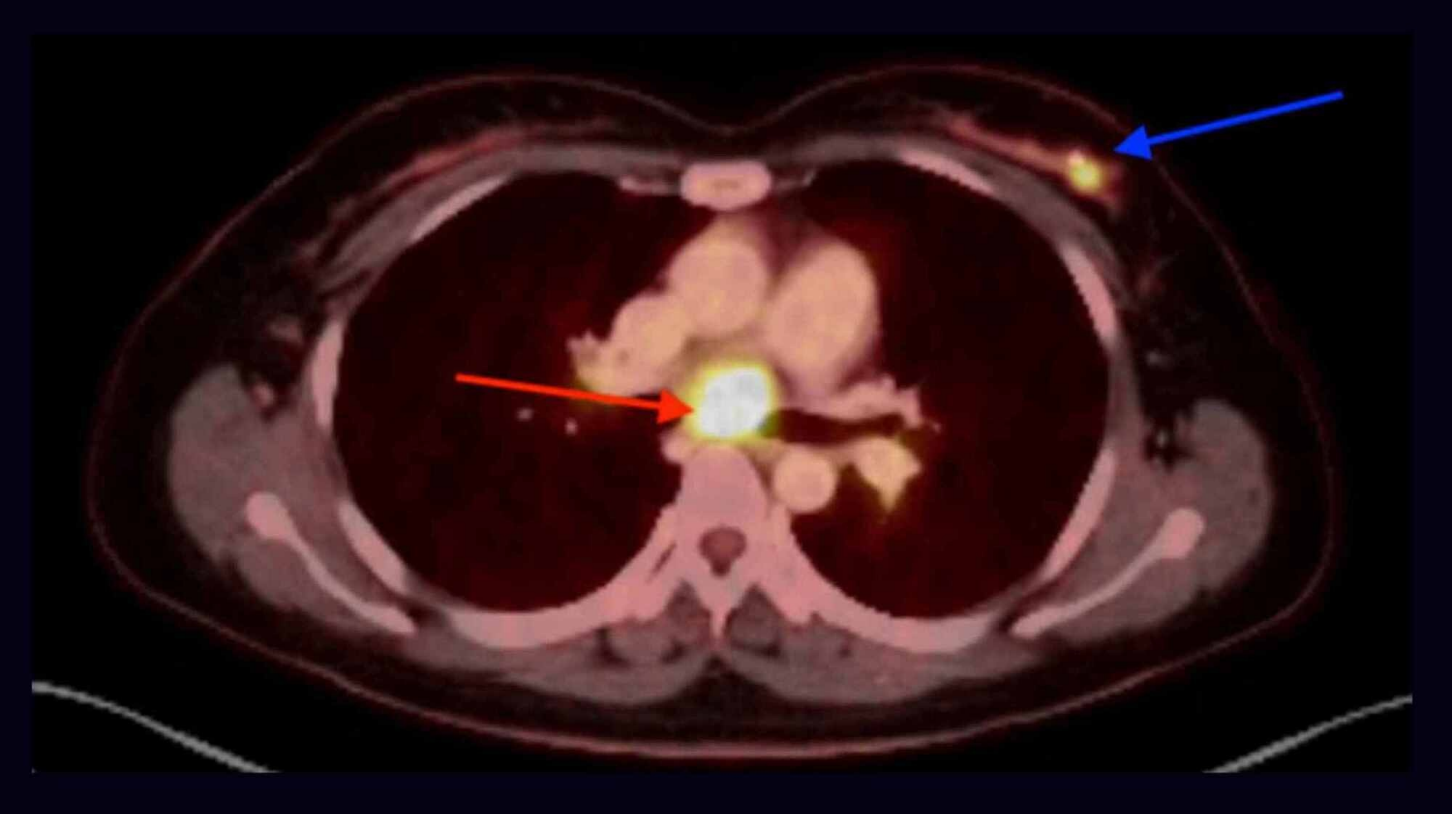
PET/CT showing FDG avid 1.2 x 3.8 x 2.1 cm mediastinal LN station 7 (red arrow) metastatic atypical carcinoid (max SUV of 12.7) and a hypermetabolic left breast mass (blue arrow) with a maximum SUV of 13 PET/CT: positive emission tomography/computed tomography; FDG: fluorodeoxyglucose; cm: centimeter; LN: lymph node; SUV: standardized uptake value
